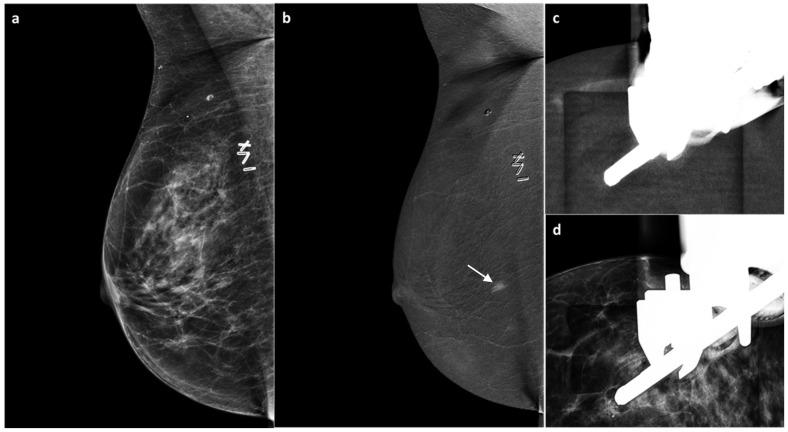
A 55-year-old BRCA-positive woman, previously treated for invasive ductal carcinoma of the right breast with partial mastectomy four years earlier. Follow-up MG revealed no suspicious findings. US demonstrated mild thickening of the surgical scar (BI-RADS 3) (a). On subsequent CEM, no enhancement was seen at the surgical scar; however, an area of non-mass contrast enhancement measuring approximately 7 mm was detected in the inner quadrants, with no mammographic or sonographic correlate (white arrow, (b)). A CEM-guided biopsy (c,d) revealed grade 2 invasive ductal carcinoma, Luminal B subtype. Bilateral mastectomy and implant reconstruction were subsequently performed.
